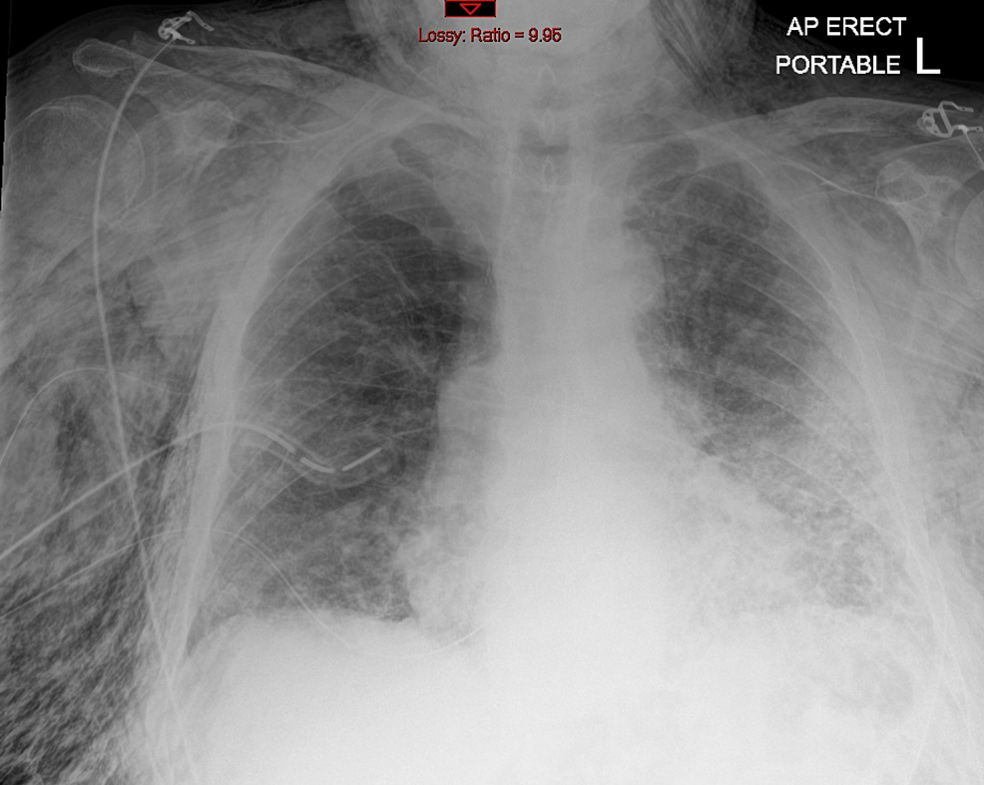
Second chest drain inserted with a subsequent decrease in the volume of the pneumothorax. Anterior-posterior (AP), portable chest X-ray.
